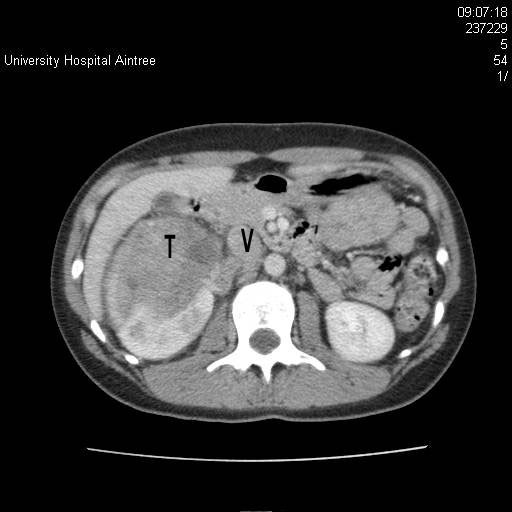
Computed tomography scan showing a large right renal tumour (T) extending into the renal vein and inferior vena cava (V).
